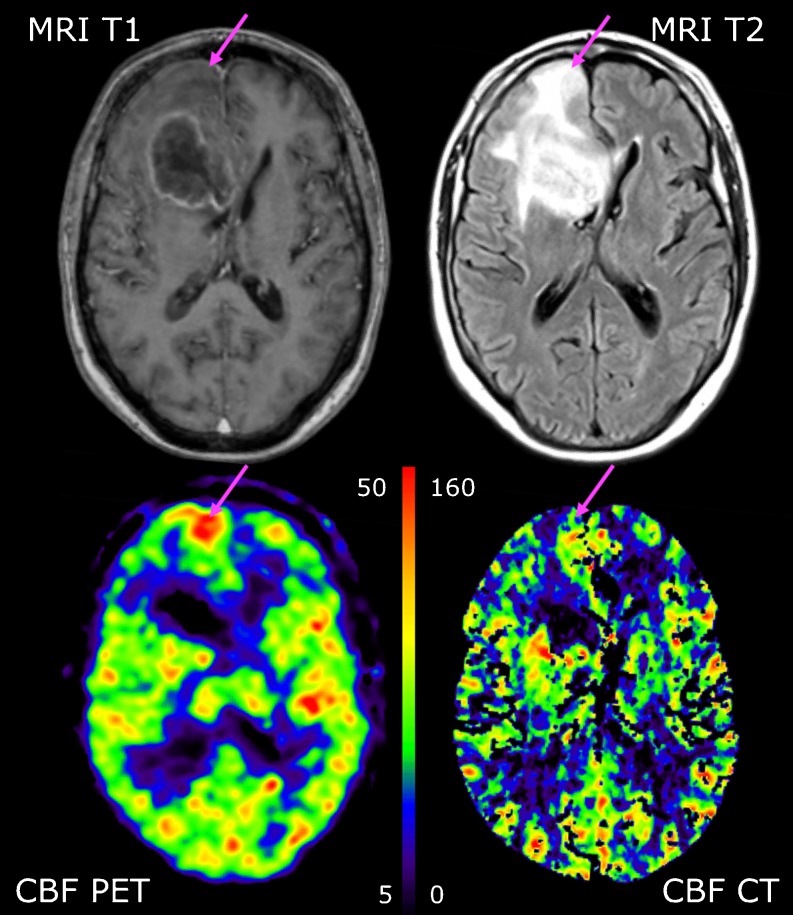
Coregistered transaxial slices of post-contrast preoperative T1-weighted MRI and FLAIR sequences (*top row*) performed 15 days prior to rCBF PET and rCBF CT scans (*bottom row*) in patient 7, suffering from glioblastoma multiforme (grade IV). The tumour has a hypoperfused cystic necrotic centre and heterogeneous hypo- and hyperperfusion in the contrast-enhancing wall. PET shows a hyperperfused area anterior to the tumour (*arrow*) that cannot be identified on rCBF CT performed within 2 h. This is likely to represent a transient non-convulsive epileptic seizure. rCBF is measured in ml min^−1^ 100 ml^−1^
